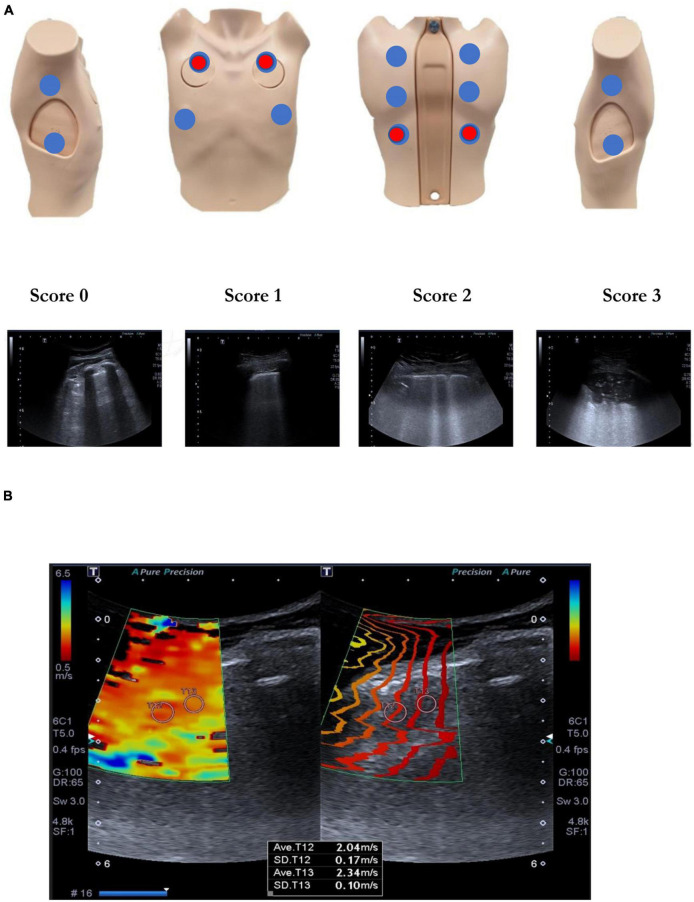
(A) Lung ultrasound. Exploration protocol of 14 areas and scoring for each area. In red the elastographic assessment points. (B) Lung 2D-SWE elastography.
